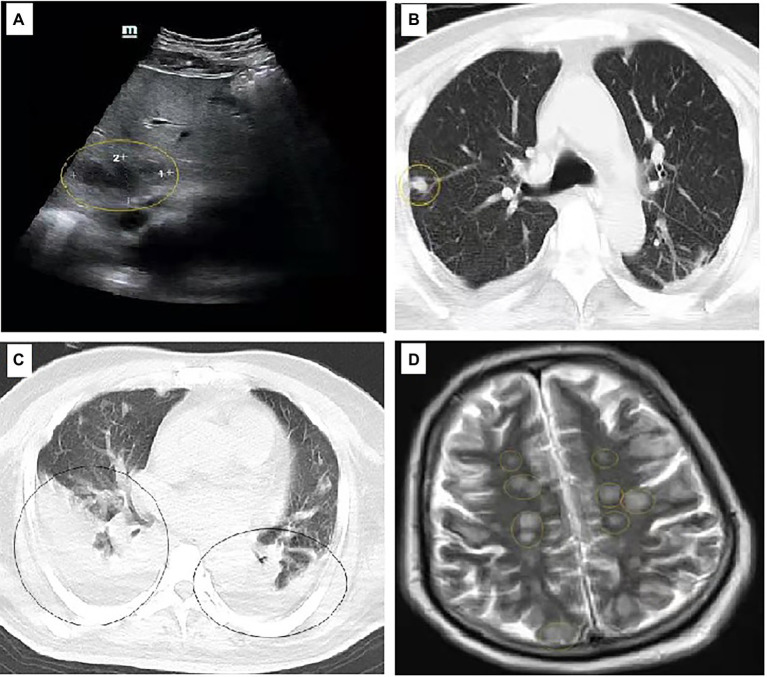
Image findings in this case. (A) Abdominal ultrasound showed the presence of intrahepatic abscess; (B,C) An computed tomography (CT) scan revealed an abscess of right lung and bilateral pleural effusion. (D) The nuclear MRI showed multiple brain abscess. Yellow circles refer to the abscesses and black circles refer to the presence of pleural effusion.
